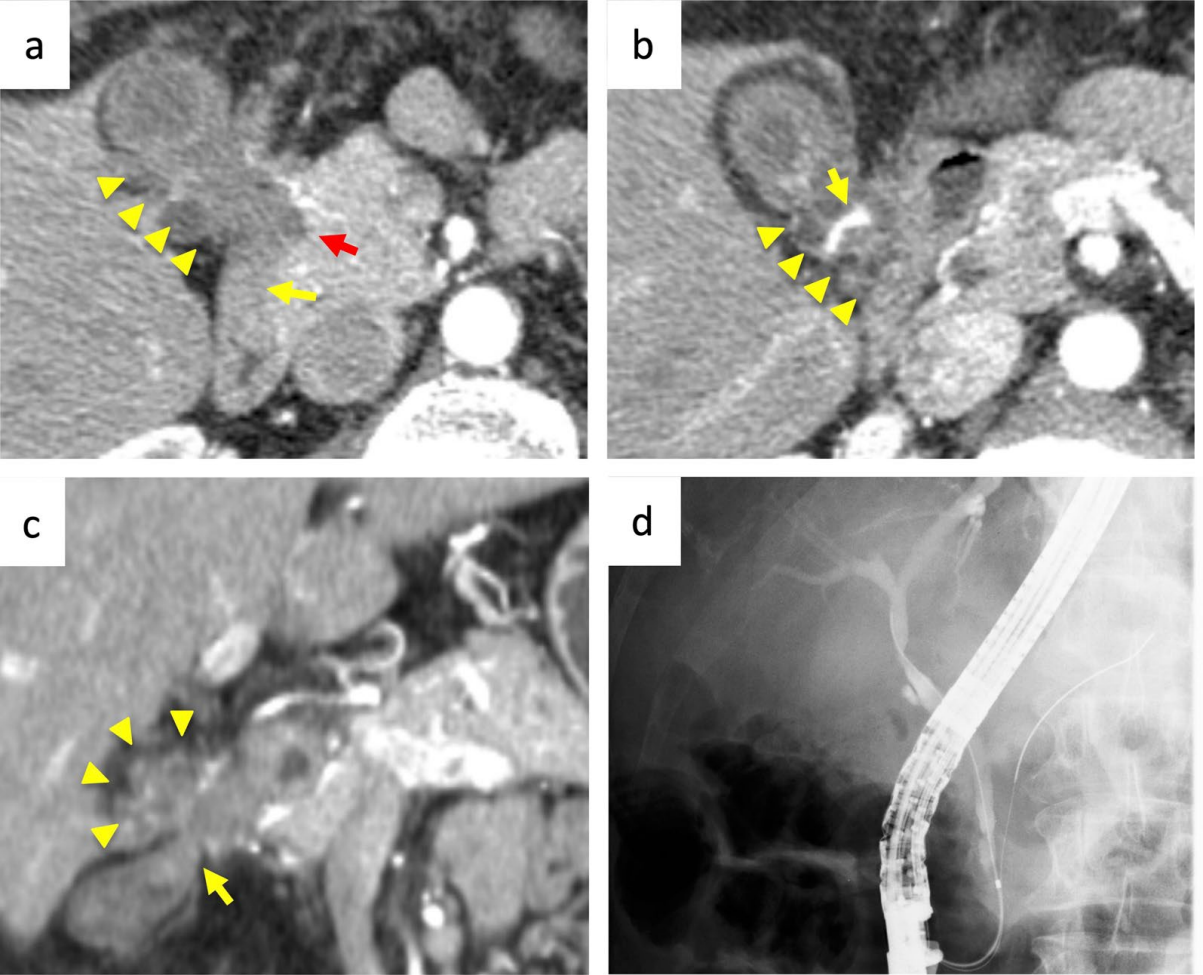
Initial radiological findings. a CT showed a gallbladder tumor (yellow arrowheads) located at the peritoneal side of gall bladder body. Infiltrations of the duodenum (yellow arrow) and the pancreas (red arrow) were also detected. b Axial image of 1.25 mm cranial side from (a). The tumor (yellow arrowheads) encased the RHA (yellow arrow). c CT coronal image showed that the tumor (yellow arrowheads) infiltrated into the hepatic flexure of the colon (yellow arrow). d Endoscopic retrograde cholangiography showed that a disruption of the cholecystic duct and the gall bladder was not contrasted, whereas the aliment of the bile duct was smooth. *CT* computed tomography; *RHA* right hepatic artery
